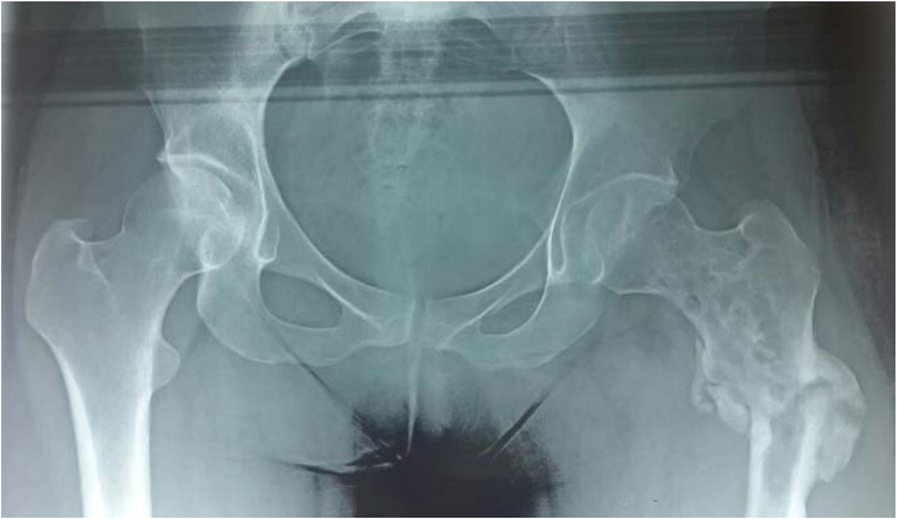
X-rays of the hip showing an osteolytic tumor at the site of the fracture (*left side*)
